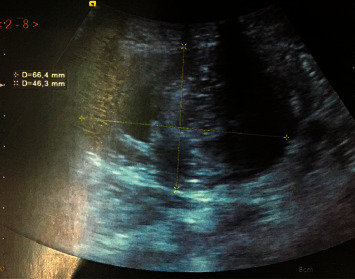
Abdominal ultrasound showing a left flank mass.
